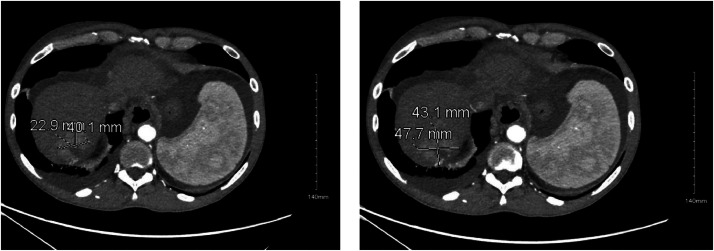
CT scan axial section, delayed phase. 2.3 cm x 4 cm lesion with early washout in Segment [VII] of liver.
